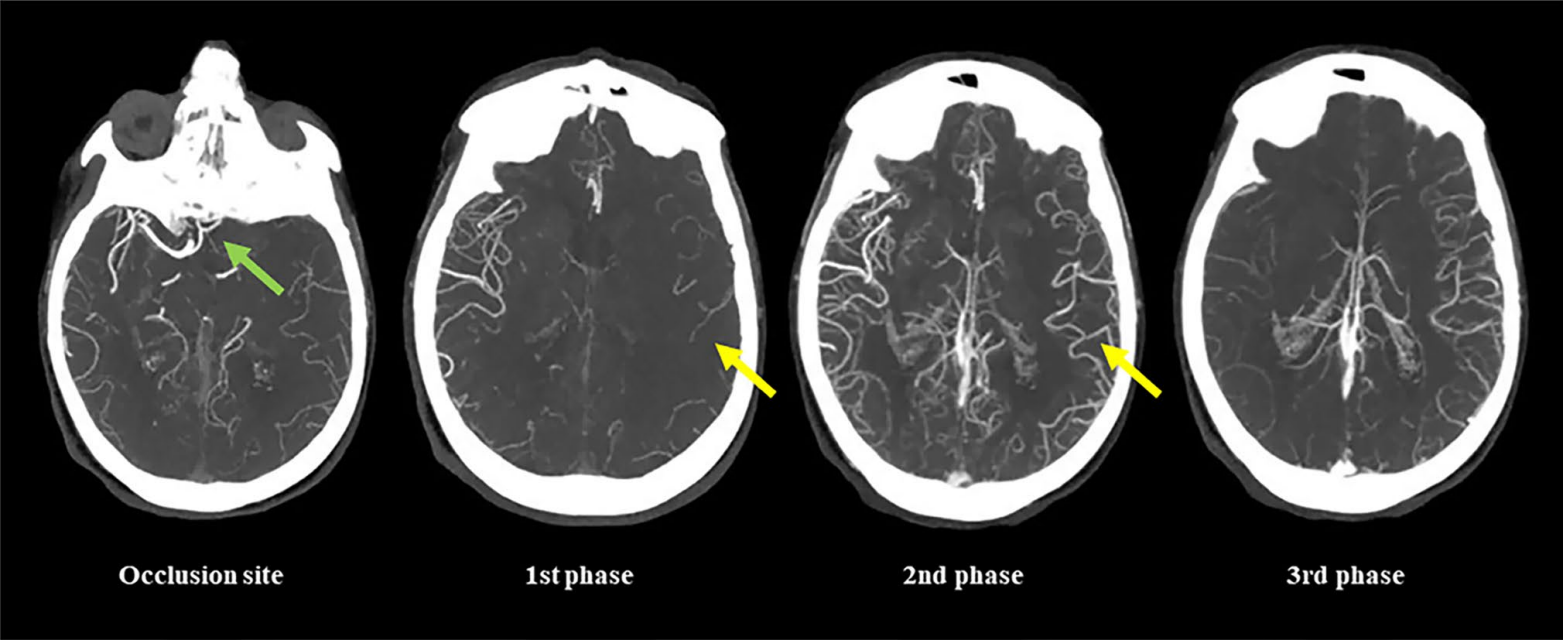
Multiphase CT angiography evaluates collaterals better than single-phase CT angiography. In the first arterial phase corresponding to single-phase CT angiography acquisition collaterals are erroneously judged as poor. On the contrary, the second peak venous phase reveals that collaterals are good. Green arrow shows occlusion site. Yellow arrows indicate collateral extent in the occluded hemisphere
